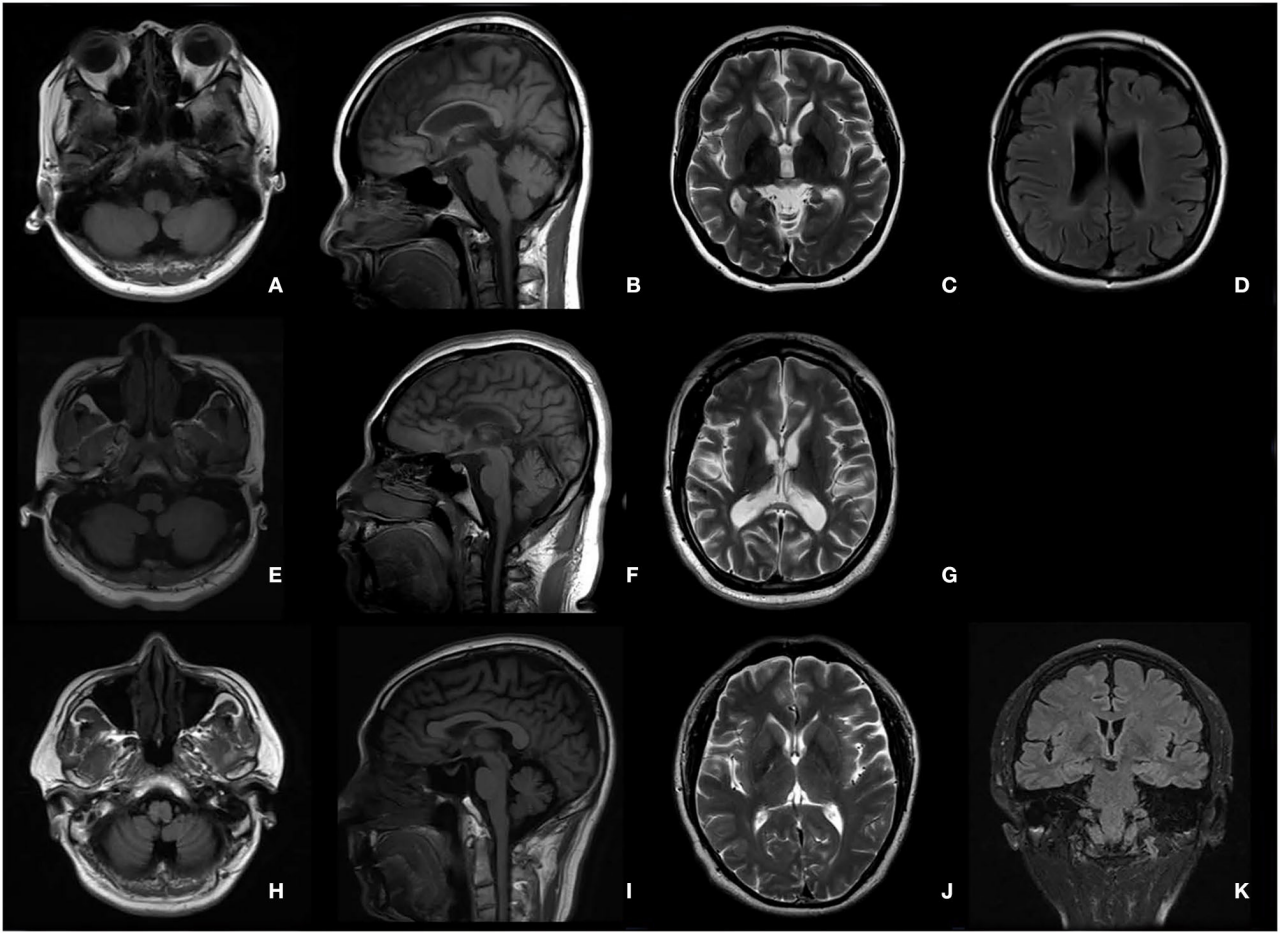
Brain MRI in patients with SPG11 (A–D), SPG15 (E–G), and SPG 5A (H–K). A thin corpus callosum (B,F), “ear of the lynx” sign (C,G), and ventricular dilation (D,G) were found in a female patient with SPG11 aged 25 years and a male patient with SPG15 aged 24 years. Periventricular white matter hyperintensities (D) are shown in the female patient with SPG11. No cerebellar atrophy was found in the patient with SPG11 (A) or in the patient with SPG15 (E). Cerebellar atrophy was the major MRI in the male patient with SPG5A aged 21 years (H,I).
